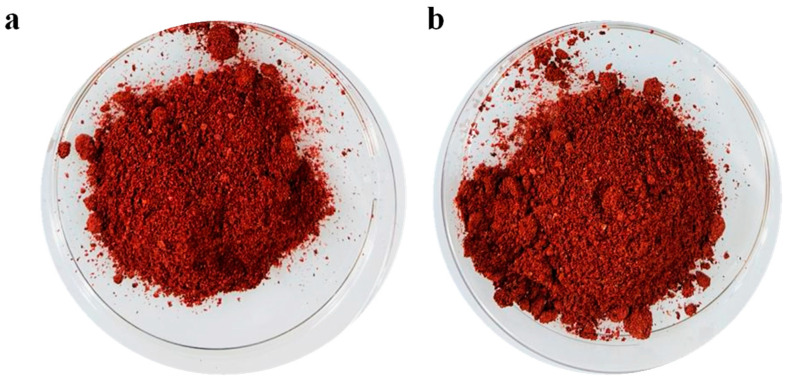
Visual characteristics of the blackberry vegetable powders analysed: (a) blackberry powder HAD-BP (hot air drying); (b) blackberry powder US-HAD-BP (ultrasound-assisted hot air drying).
